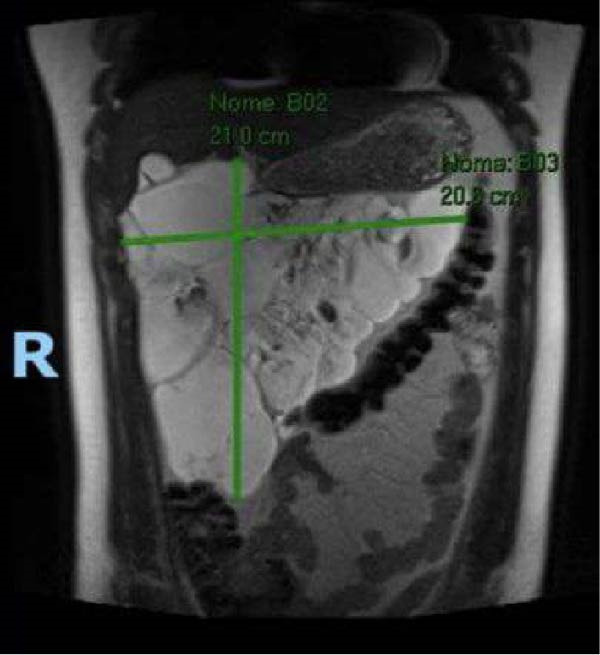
Coronal T2‐weighted MRI demonstrating a multiloculated cystic lesion measuring 21.0 cm × 20.8 cm within the abdominal cavity, characterized by thin internal septations, a thin and smooth wall, and homogeneous hyperintense fluid content. The lesion exerted a significant mass effect on the adjacent bowel loops and liver. The imaging findings were consistent with cystic lymphangioma.
